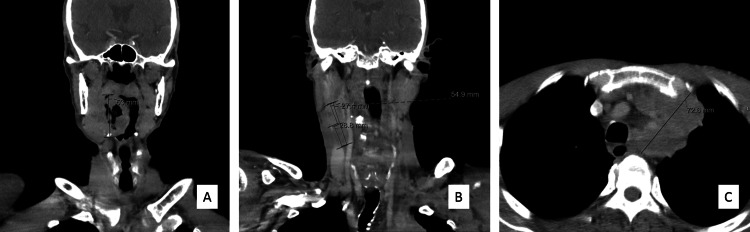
Pharyngeal-neck and thorax computed tomography scans (A) Coronal view of contrast-enhanced pharyngeal-neck computed tomography (CT) scan showing an expansive and infiltrative lesion on the right lateral wall of the oropharynx that extends from the plane of the soft palate to the base of the tongue and pharyngoepiglottic fold, reaching a maximum longitudinal axis of 53 mm (ruler). The lesion invades the root of the tongue and the sublingual space; (B) Coronal view of contrast-enhanced pharyngeal-neck CT revealing two coalescing adenopathies on the right involving levels II and III and reaching 55 mm (ruler) in the longest longitudinal axis - the uppermost solid and measuring 27 mm (ruler) and the lower, predominantly necrotic, measuring 29 mm (ruler) in the longest axis; and (C) Axial view of contrast-enhanced staging thorax CT revealing mediastinal involvement with heterogeneous adenopathies containing central areas of necrosis - the most expressive conglomerate surrounds the supra-aortic trunks, without a cleavage plane with the aortic arch, with a 73 mm longest axis (ruler) and causing a deviation of the trachea to the right
